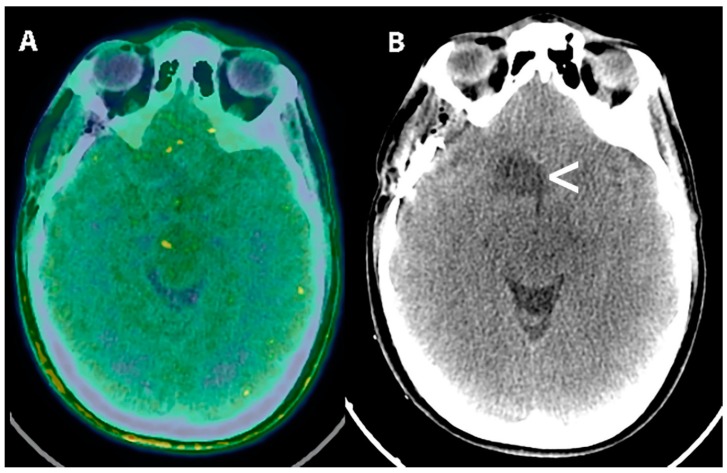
[^18^F] FDOPA PET (A) and T2 FLAIR MRI (B) in a 14-year-old female patient with the suspect of primary brain tumor. T2 FLAIR image showed an area of mild hyperintensity in the left temporal lobe(B,<). [18F] FDOPA PET/CT scan demonstrated increased uptake of the radiopharmaceutical in the lesion with a Standardized uptake value equal to 2.2 vs. 1.1 of the surrounding brain tissue (A,<). The patient was surgically treated and the subsequent diagnosis of low-grade glioma (WHO grade II).
